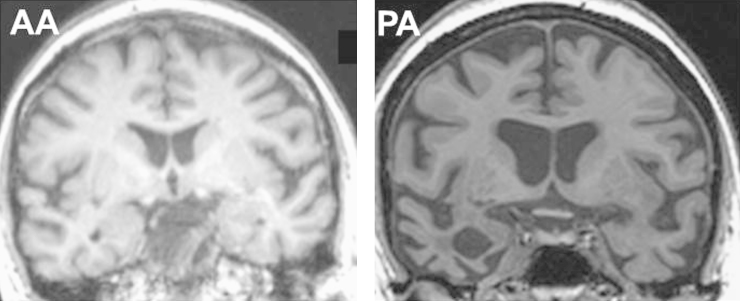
Representative coronal T1-weighted MR images from the patients (left hemisphere shown projected on the right side in each case): AA (left), showing asymmetric predominantly left-sided peri-Sylvian atrophy; and PA (right), showing asymmetric, predominantly right-sided anterior and mesial temporal lobe atrophy.
